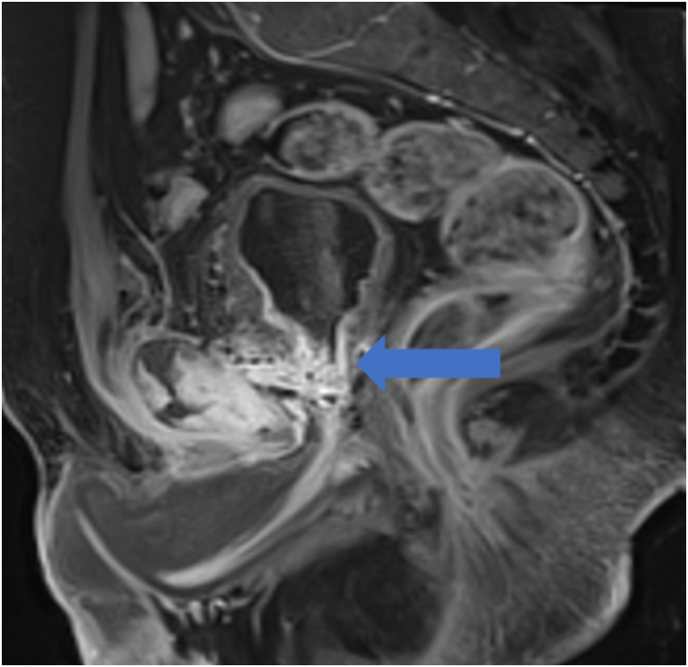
Post-contrast SAG T1-weighted MRI sequence showing communication between the bladder and symphysis with patological enhancement and abscess formation.
